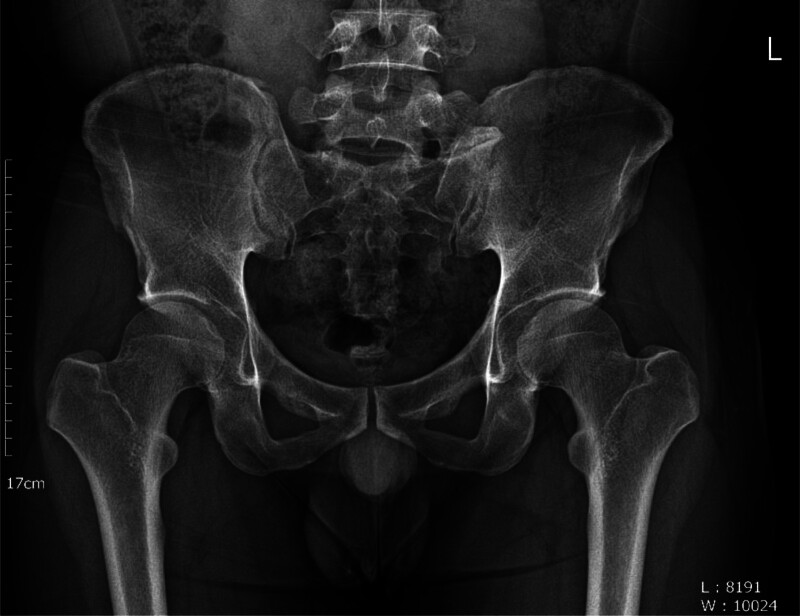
Radiographic findings depicting Bertolotti syndrome.
